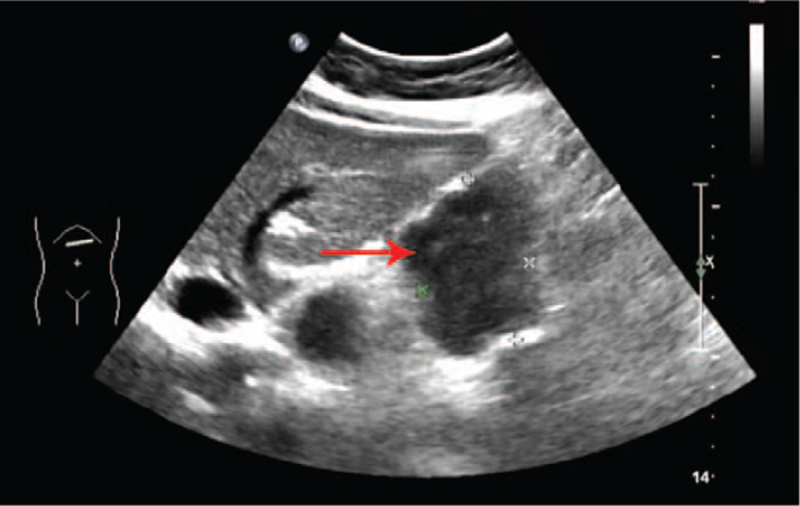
Ultrasound findings. Ultrasound revealed a 5.6 × 3.6 cm, inhomogeneous hypoechoic well-defined lesion (red arrow) in the pancreatic tail.
